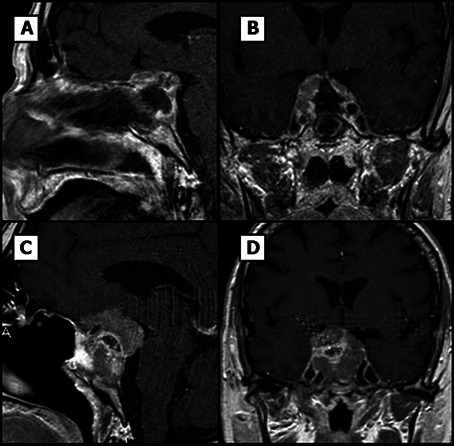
Pituitary MR images. (A, B) Sagital and coronal T1-weighted postsurgical images. (C, D) Sagital and coronal T1-weighted images, tumor rest growing can be noticed from small capsular rest to 20 mm invasive tumor.
